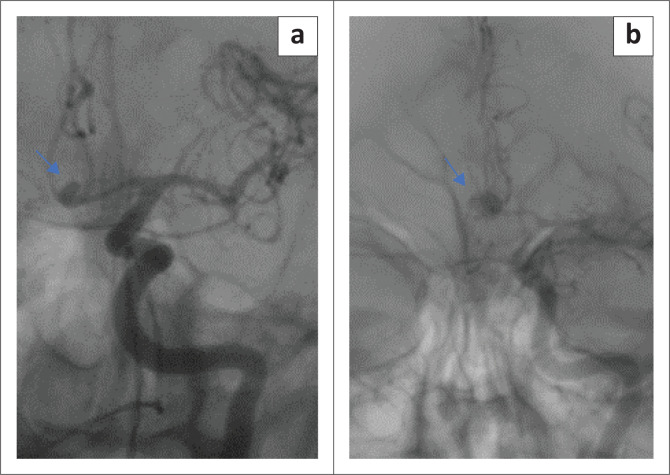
Left internal carotid artery angiogram (non-subtracted images) frontal views of different patients. (a) Very small (2.8 mm) saccular aneurysm arising from the anterior communicating artery (arrow), which was missed on computed tomography angiography, in a 62-year-old male. (b) Small (4.4 mm) saccular aneurysm also arising from the anterior communicating artery (arrow), which was correctly identified on computed tomography angiography, in a 54-year-old female.
